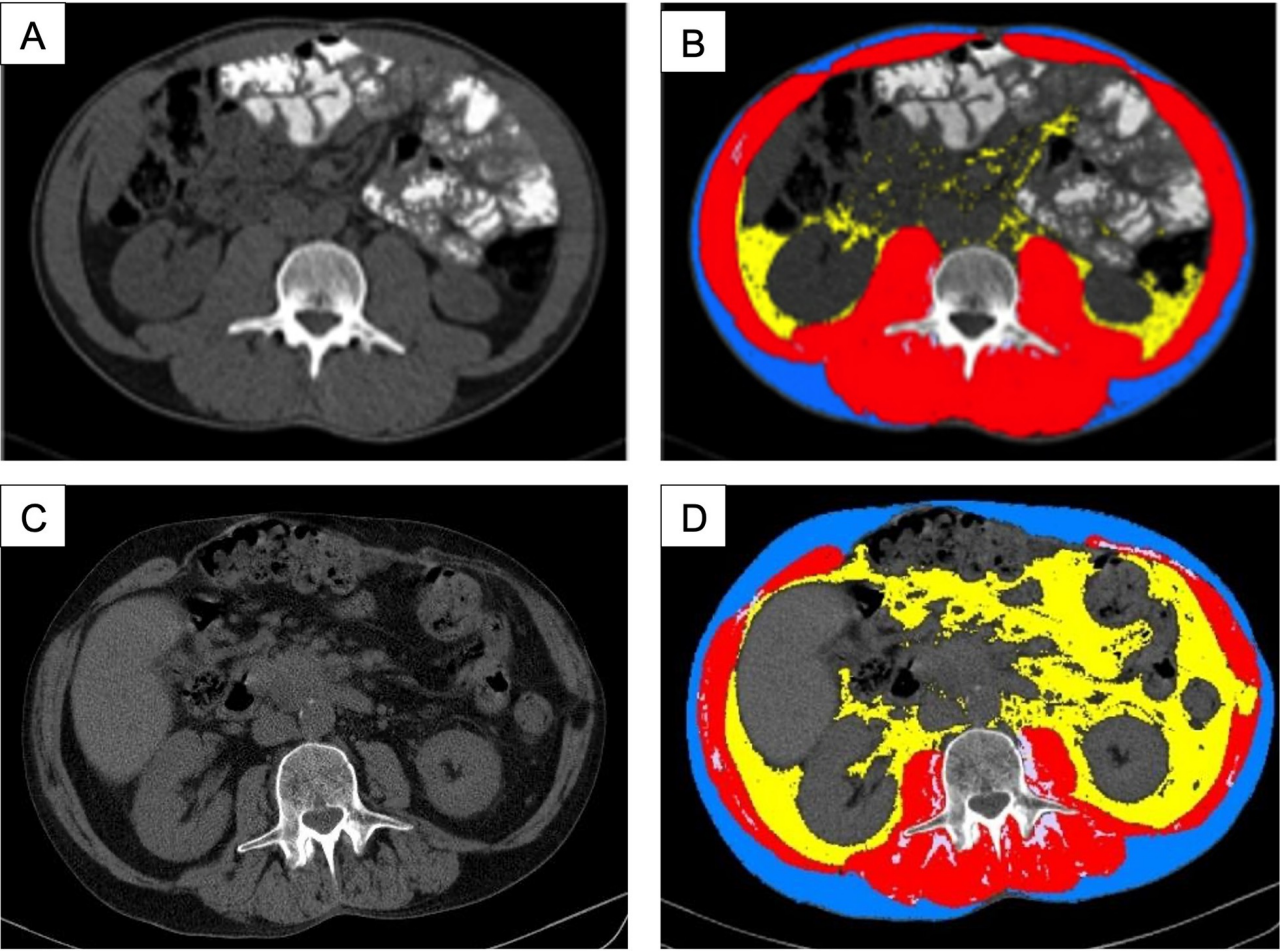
Axial CT-scans of two different patients with a healthy BMI value of 22.1 kg/m^2^. A and B: a patient without sarcopenia, both BMI and SMI are within the normal range, 22.1 kg/m^2^ and SMI 53.5 cm^2^/m^2^, respectively. A—initial CT-scan on the level of L3 vertebral body; B—segmented CT scan; the area of muscle tissue was 179.2 cm^2^; the total area of fatty tissue was 102.5.2 cm^2^.BA C and D: a patient with sarcopenia, BMI was normal with 22.1 kg/m^2^, SMI was decreased with 36.2 cm^2^/m^2^.DC A—initial CT image, performed at the L3 vertebral body level; B—segmented CT tomogram; the area of muscular tissue was 122.1 cm^2^; the total area of fatty tissue was 232.2 cm^2^.
